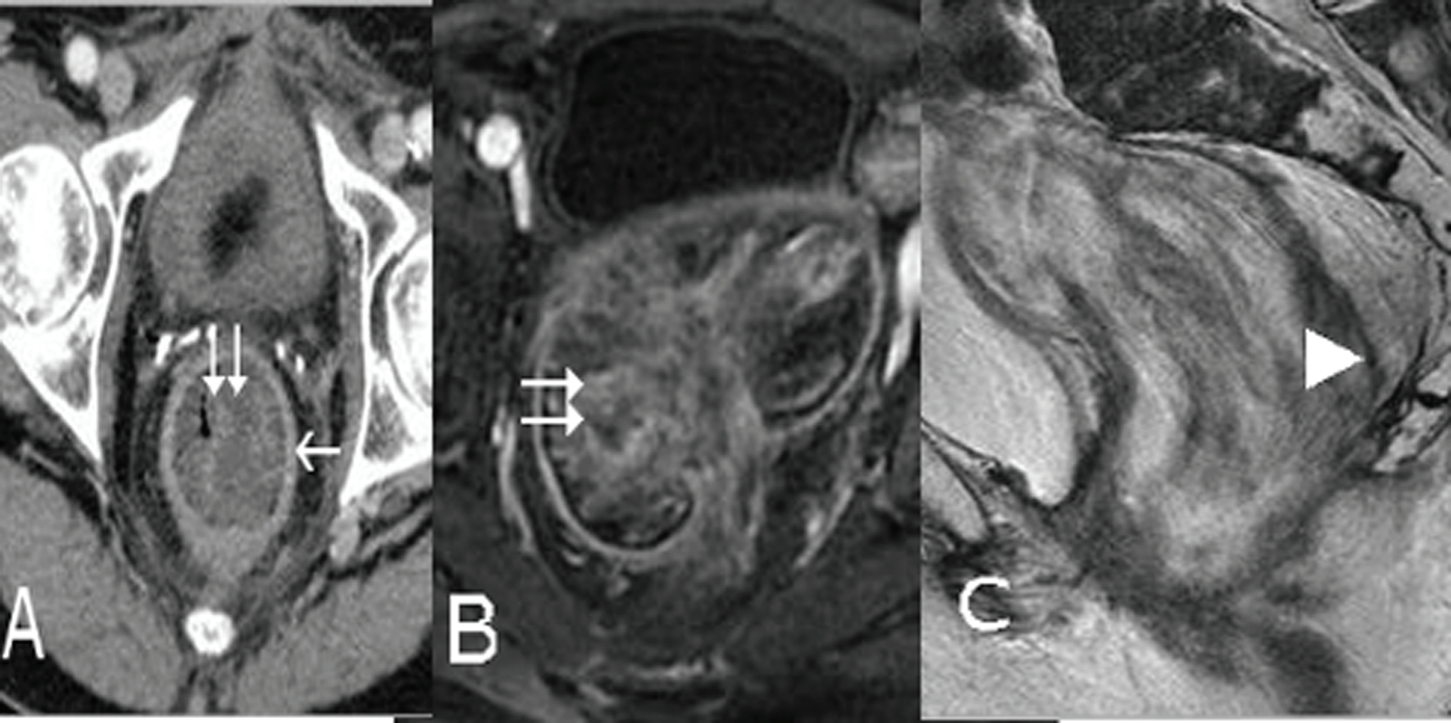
A) Transversal view of abdominal CT scan. Tumor filling of the rectal lumen (double arrow) and circumferential thickening of the rectal wall (arrow). B) RMI, T2-weightened sequence. Heterogeneous, hyper-signal tumor filling the whole rectal lumen (double arrow). C) RMI, T1-weightened sequence, sagittal view. Invasion of the posterior rectal wall (arrow point).
